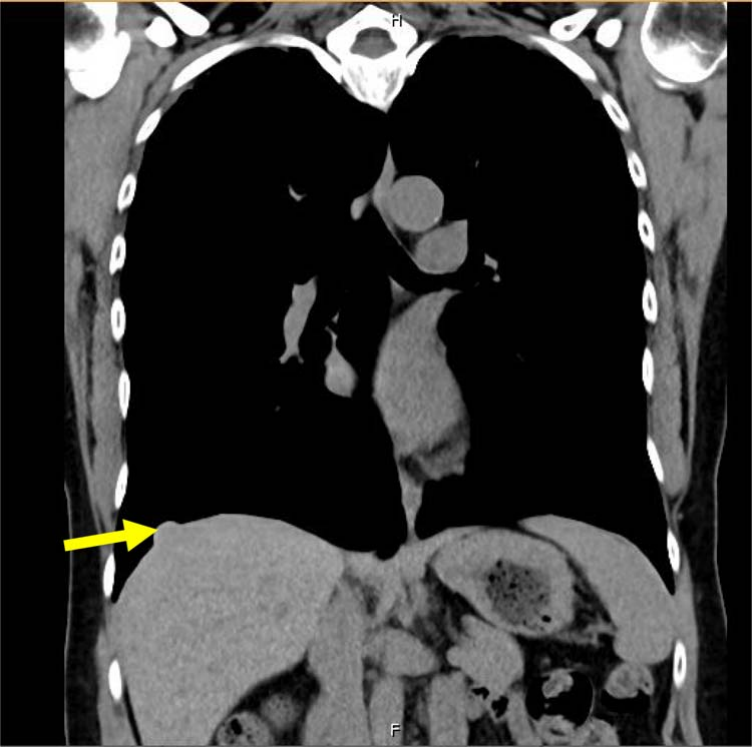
Smaller nodule measuring 0.9 cm × 1.5 cm on CT scan.
